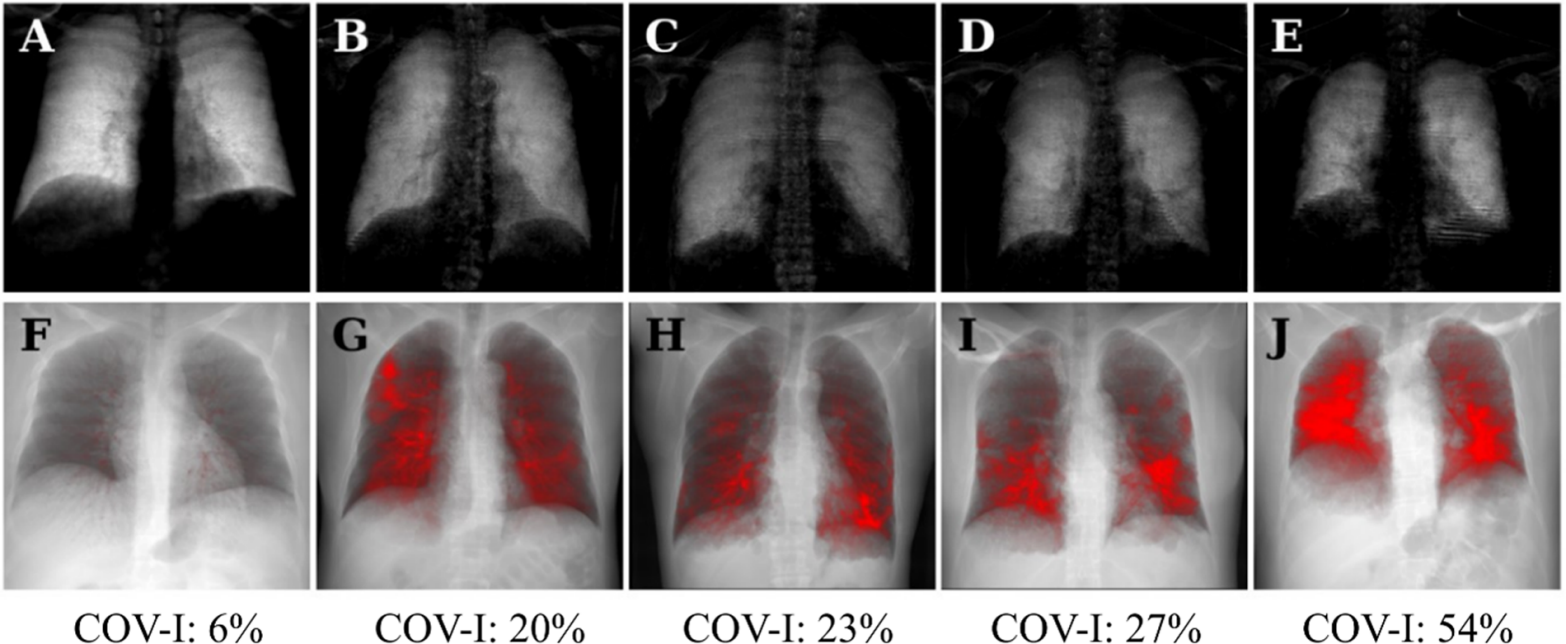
Dark-field radiographs (A–E) and projections of CT-based COVID-19 pneumonia quantification (F–J) in a healthy, 30-year-old man (A,F) and four patients (three men, one woman) with COVID-19 pneumonia (B–E, G–J); respective COVID-19 index below. The same window and level settings were applied within each modality. Conventional radiographs reveal typical opacities in lung regions affected by COVID-19-associated changes, such as predominantly in the right upper field in the first COVID-19 patient (B). While the dark-field chest radiograph of the healthy subject exhibits a strong homogeneous dark-field signal (A), the dark-field signal intensity of the patients with COVID-19 pneumonia appears decreased overall and exhibits a regionally inhomogeneous patchy pattern (B–E), corresponding well to the affected areas in the CT-based projections (red overlay, G–J). The red overlay in the CT-based projection of the healthy participant (F) corresponds to the bronchovascular bundle.
